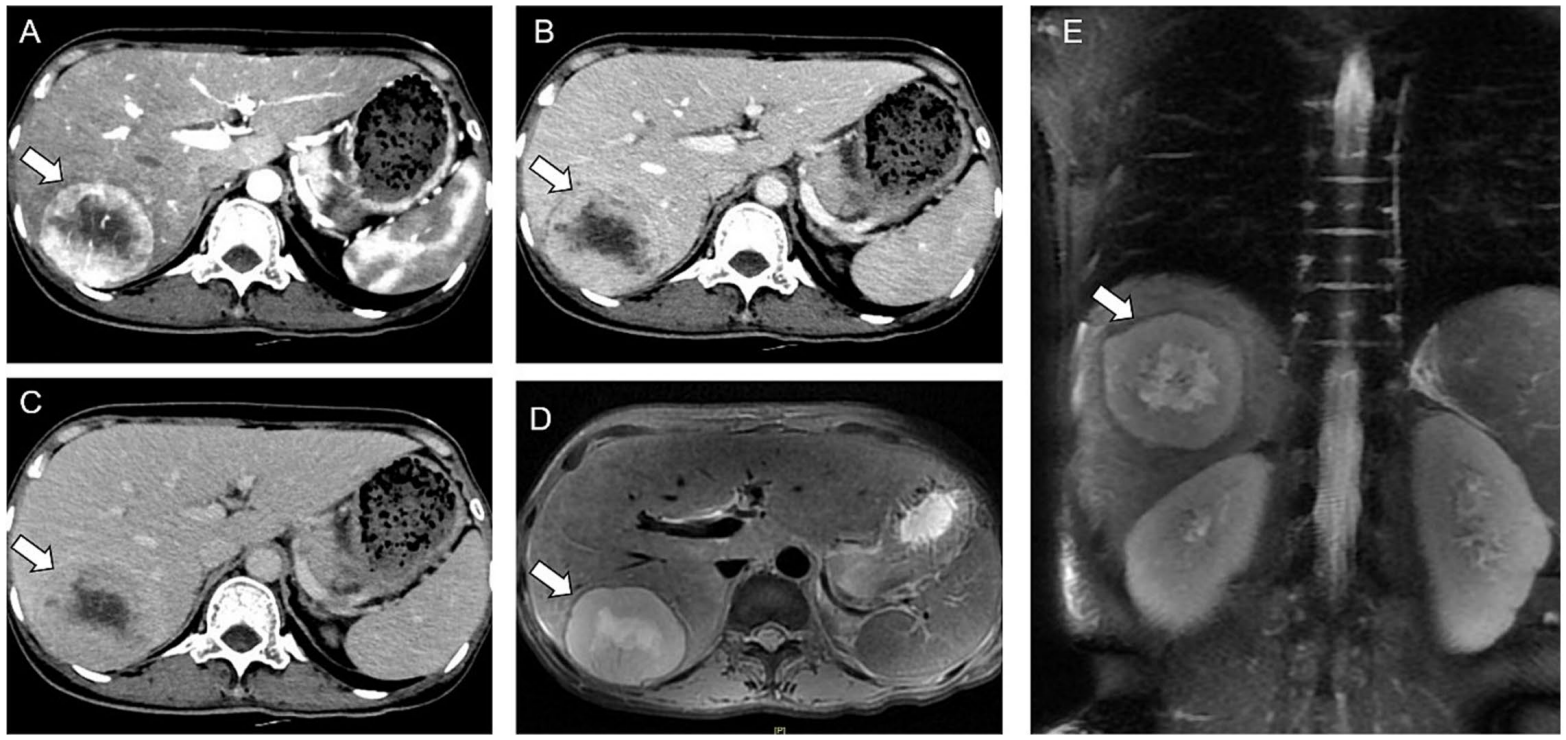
Computed tomography (CT) and magnetic resonance imaging (MRI) results. (A–C) Arterial, venous, and delayed phases of enhanced CT. (D,E) MRI shows a huge round mass.
